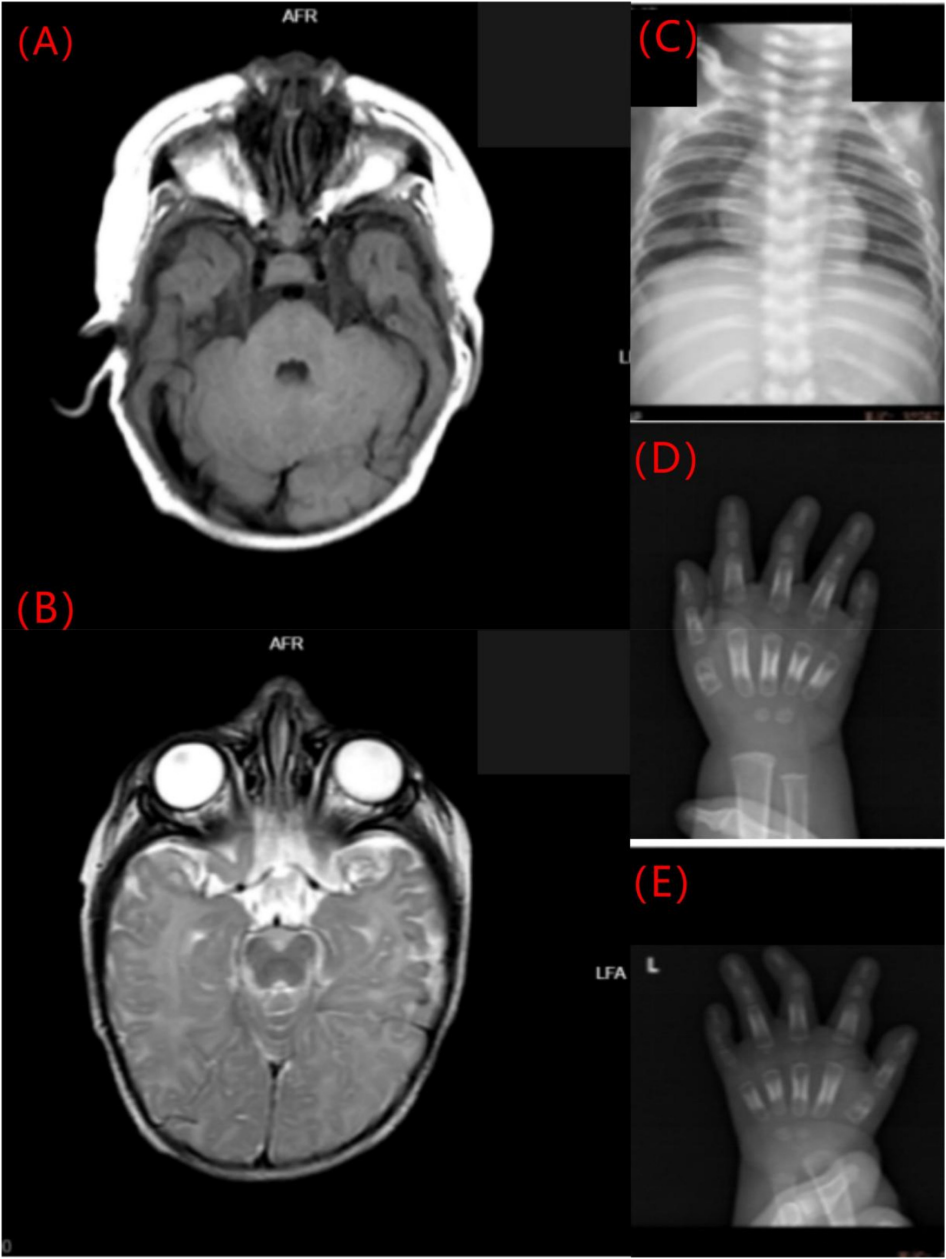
Imaging findings of the LMS patient. (A) Mild hemosiderin deposition in the left cerebellar hemisphere (axial MRI). (B) Focal cerebral atrophy with surface hemosiderin deposits in the left frontal lobe (axial MRI). (C) Thickened chest ribs and cortical bone (anteroposterior radiograph). (D) Deformed right-hand phalanges with cortical thickening and irregular contours (anteroposterior radiograph). (E) Abnormal morphology and cortical thickening of the middle phalanges of the left hand (anteroposterior radiograph).
